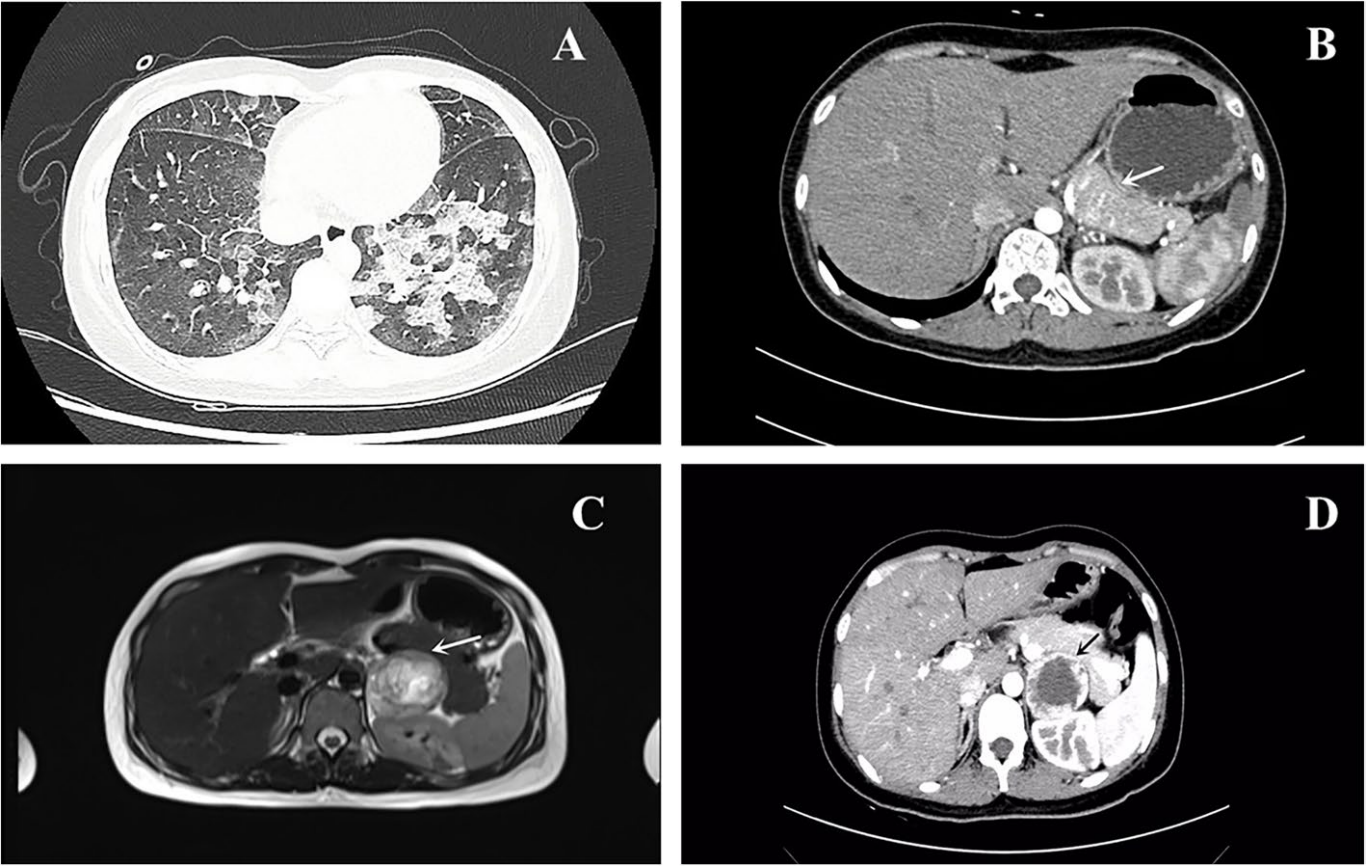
A Chest computed tomography scan showing bilateral pulmonary edema. B Abdominal CT scan: a left adrenal mass measuring 4 × 5 cm. C Adrenal MRI: a left adrenal tumor measuring 5.6 × 4.6 cm with hemorrhage. D Enhanced adrenal CT showing a left adrenal mass with an uneven density measuring 4.8 × 4.1 cm
